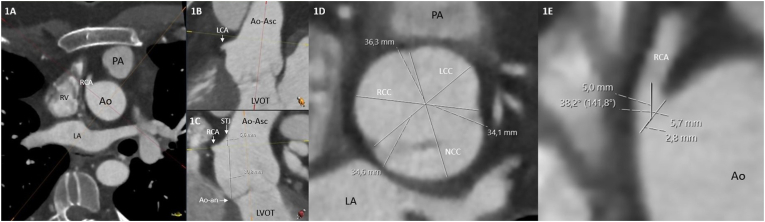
CTA analysis approach. A–C: 3-dimensional multiplanar reconstruction with double oblique planes perpendicular to the neo-aortic annulus. C: 3-dimensional multiplanar reconstruction plane to additionally measure the distance from the coronary ostium to the neo-aortic annulus. D: Cusp-to-commissure diameter measurements of the neo-aortic root. E: Measurement of the coronary take-off angle. Abbreviations: Ao, aorta; Ao-an, neo-aortic annulus; Ao-Asc, ascending aorta; CTA, computed tomography angiography; LA, left atrium; LCA, left coronary artery; LCC, left coronary cusp; LVOT, left ventricular outflow tract; NCC, non-coronary cusp; PA, pulmonary artery; RCA, right coronary artery; RCC, right coronary cusp; RV, right ventricle; STJ, sinotubular junction.
